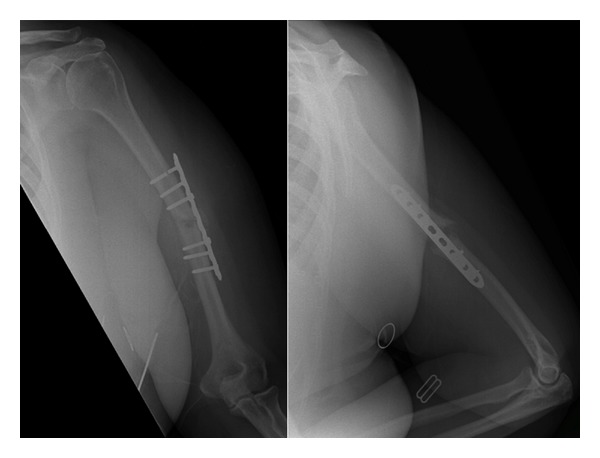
AP and lateral radiographs demonstrating proximal screw breakage and oligotrophic nonunion.
